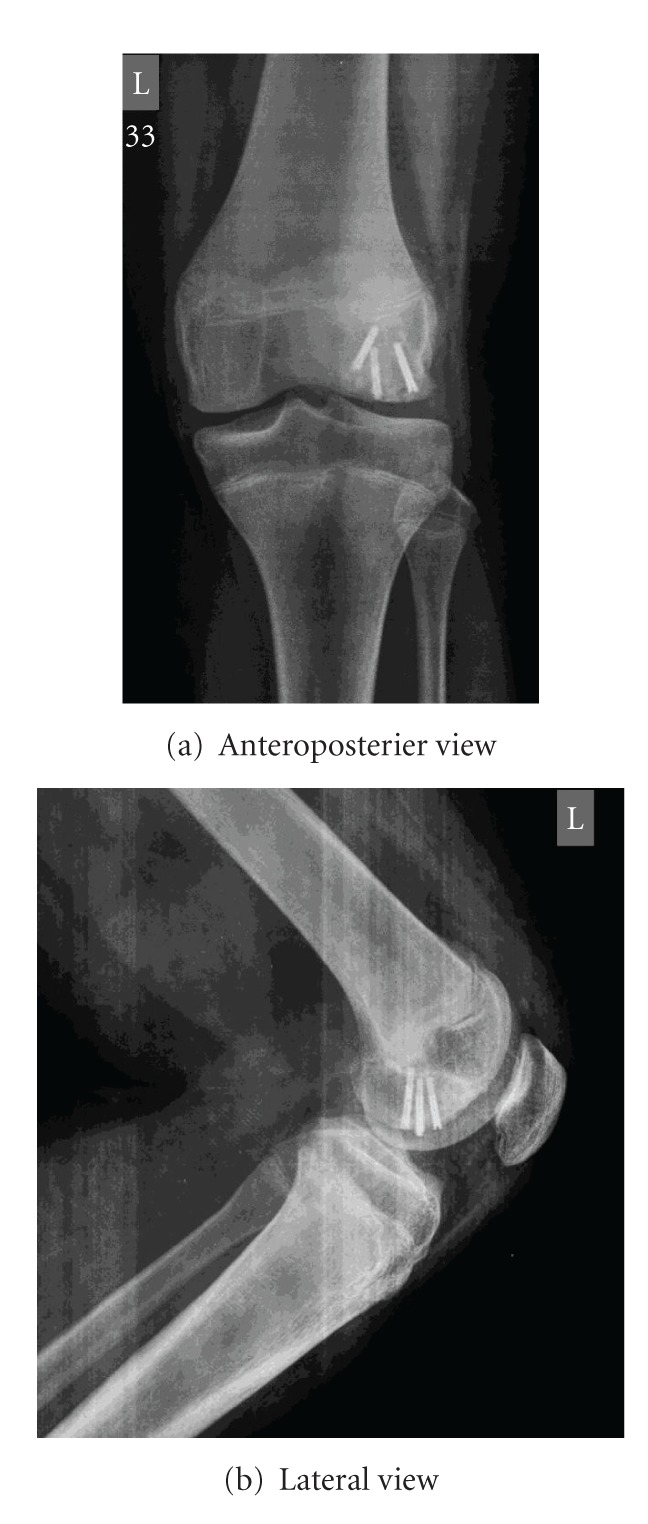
Radiograph at one year follow-up.
